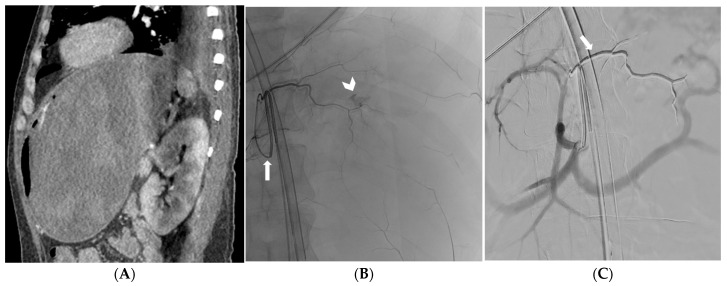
CT angiography reformatted image in the oblique sagittal plane depicting recurrent massive gastric bleeding after endoscopic treatment (A). Selective catheterization of the left gastric artery (arrow) with active bleeding confirmed at fluoroscopy (arrowhead) (B). Digital subtraction angiography showing effective embolization and Onyx 18 cast (arrow) distributed along the left gastric artery (C).
